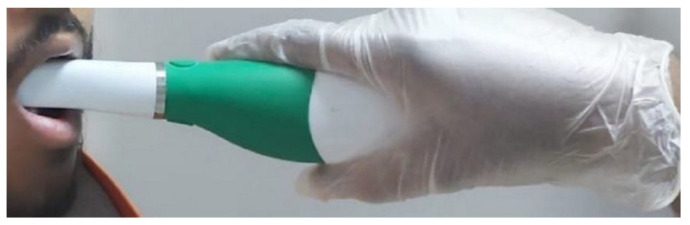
Intra-oral ultrasonography.
